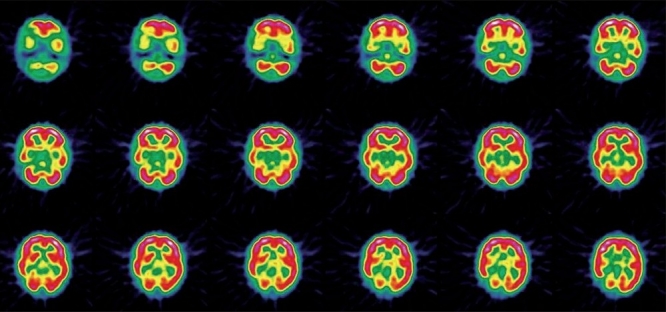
Postoperative SPECT imaging showing hypoperfusion in the right temporo-occipital area suggestive of an old infarct. There is evidence of no other perfusion defects seen in the rest of the brain parenchyma.
